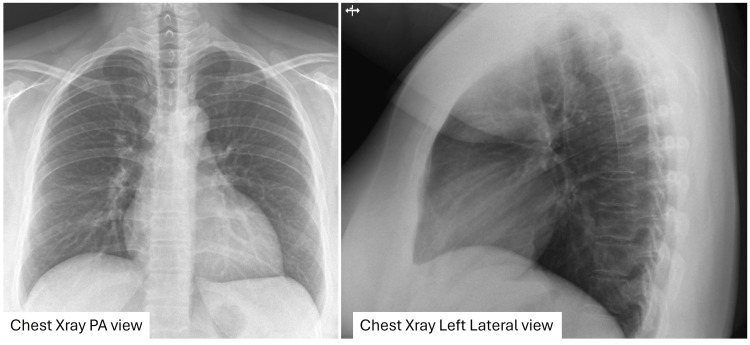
Chest X-ray PA and left lateral views showing mild cardiomegaly No evidence of pulmonary pathology was identified. PA, posteroanterior.
